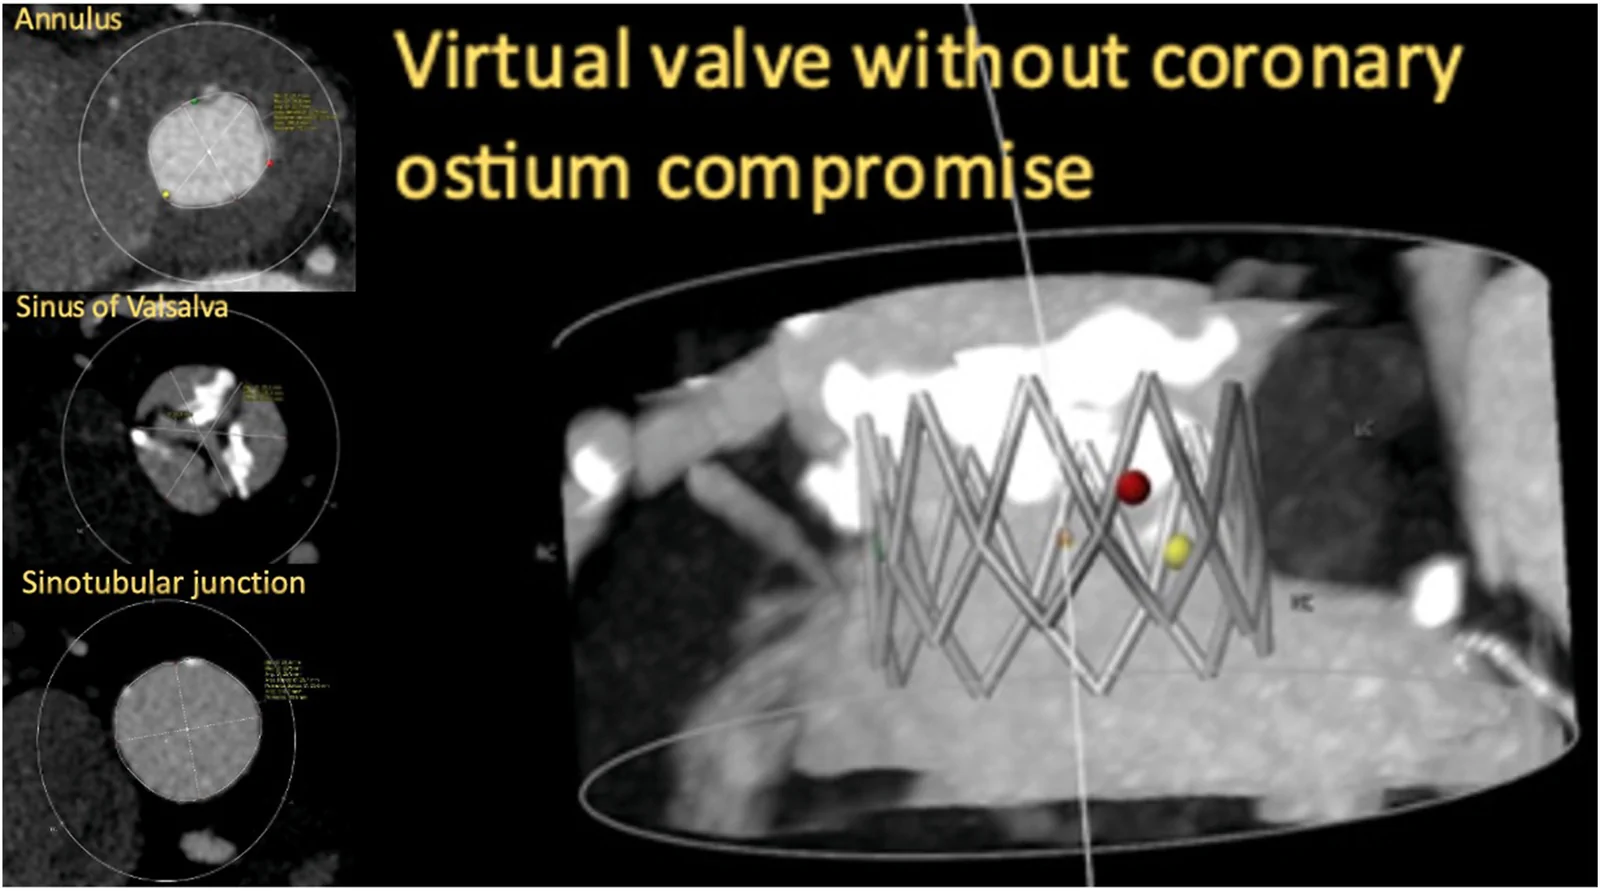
Computed tomography analysis.
